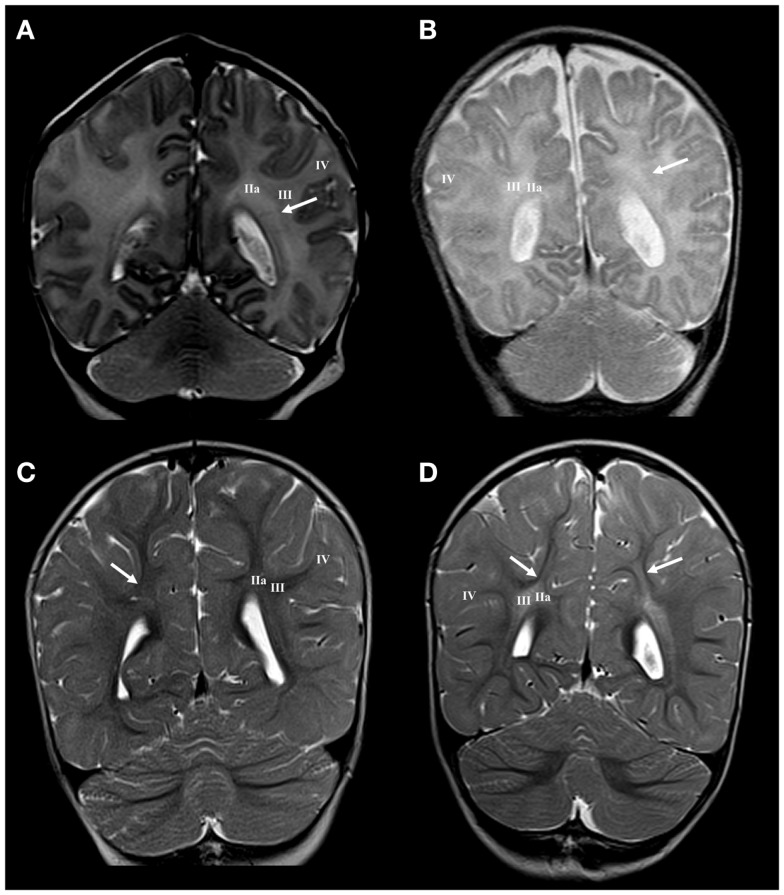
Longitudinal MRI follow-up of centrum semiovale perinatal lesion on coronal T2 images. Normal findings of centrum semiovale at term age (A) with visible border between parietal crossroad and centrum semiovale [arrow in (A)]. Normal findings at the age of 13 months with barely visible border between U-fibers and centrum semiovale [arrow in (C)]. Term born child (BW = 3960 g, SNAPII = 40, SNAPPEII = 58, AS = 0/4) with perinatal asphyxia and diffuse hyperintensity of white matter with diminished border between parietal crossroad and centrum semiovale at term equivalent age [arrow in (B)] but with enhanced visibility of border between U-fibers and hyper-intensive centrum semiovale at the age of 13 months [arrows in (D)]. Numbers I–IV represent segments of white matter as previously described.
